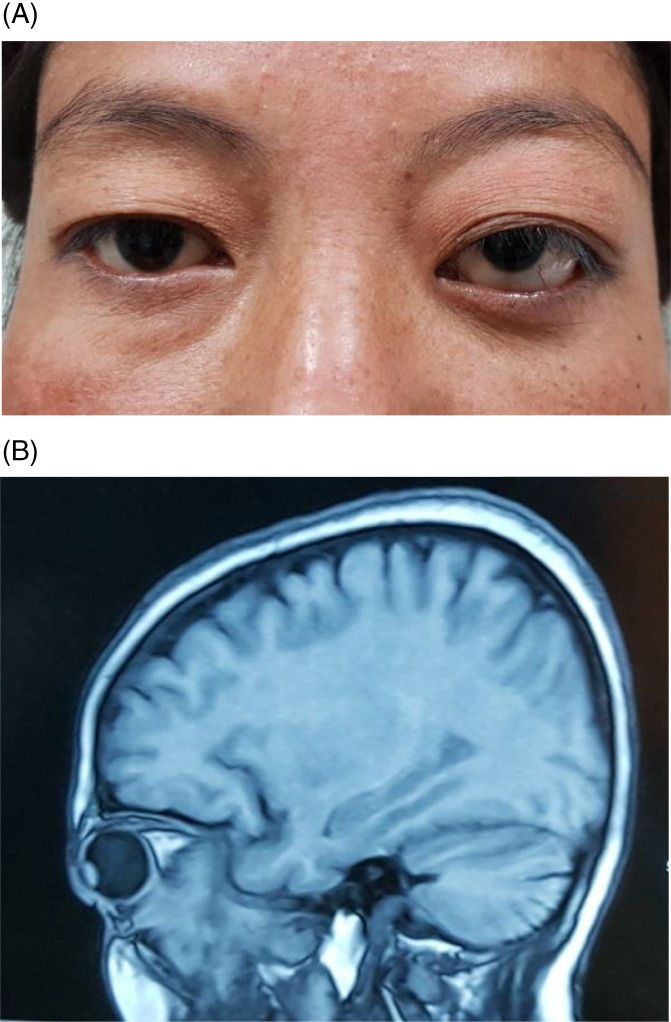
Sinonasal carcinoma invading left orbit. (A) Presenting as proptosis. (B) MRI image showing maxillary carcinoma invading the floor and retrobulbar orbit
